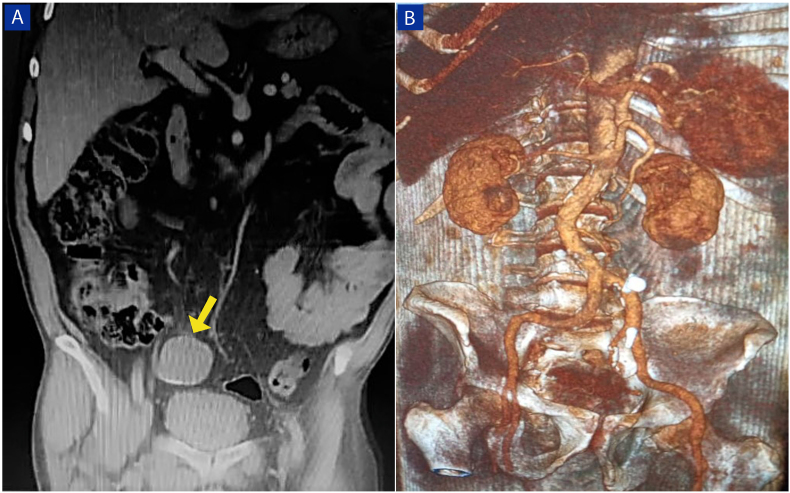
(A) Coronal contrast-enhanced CT image shows a heterogeneous mass in the right lower quadrant in the expected location of the appendix with tiny peripheral calcifications (yellow arrow), associated with bladder tumor. (B) A three-dimensional CT angiographic image demonstrates the integrity of the right common iliac artery. . (For interpretation of the references to colour in this figure legend, the reader is referred to the Web version of this article.)
